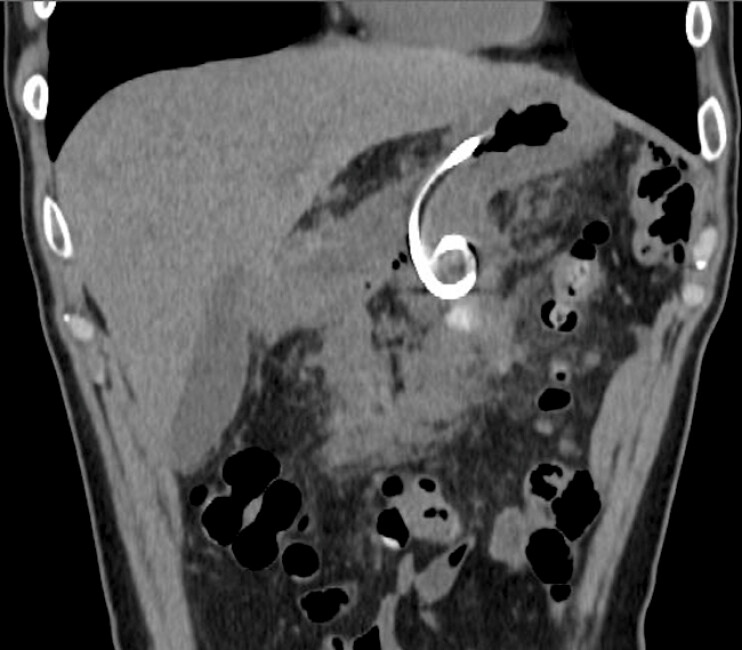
Computed tomography showed a successfully deployed 7-Fr double pigtail plastic stent.
